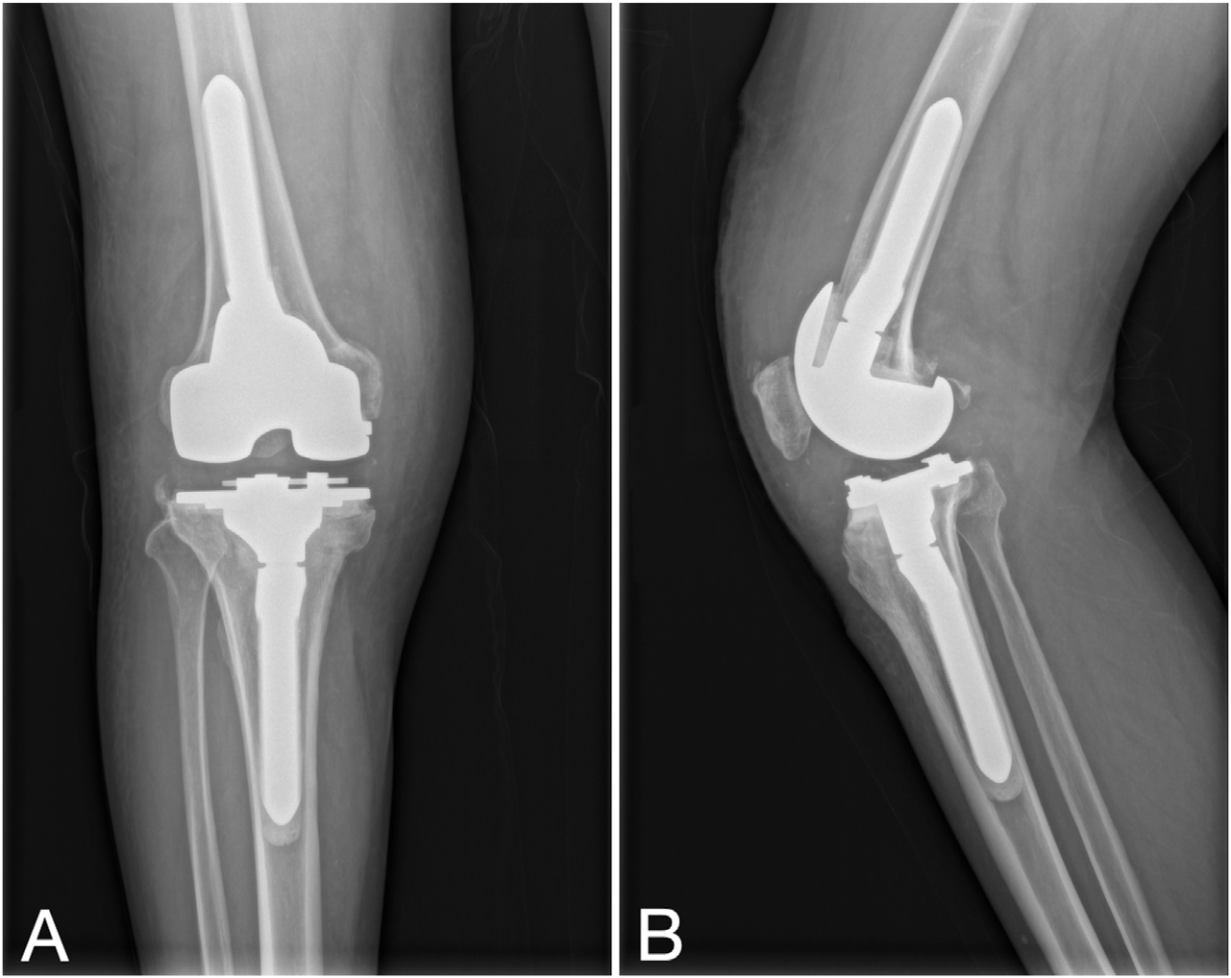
Second-stage procedure with implantation of a new revision prosthesis. (A, B) Knee X-rays depict the prosthesis in a satisfactory position.
